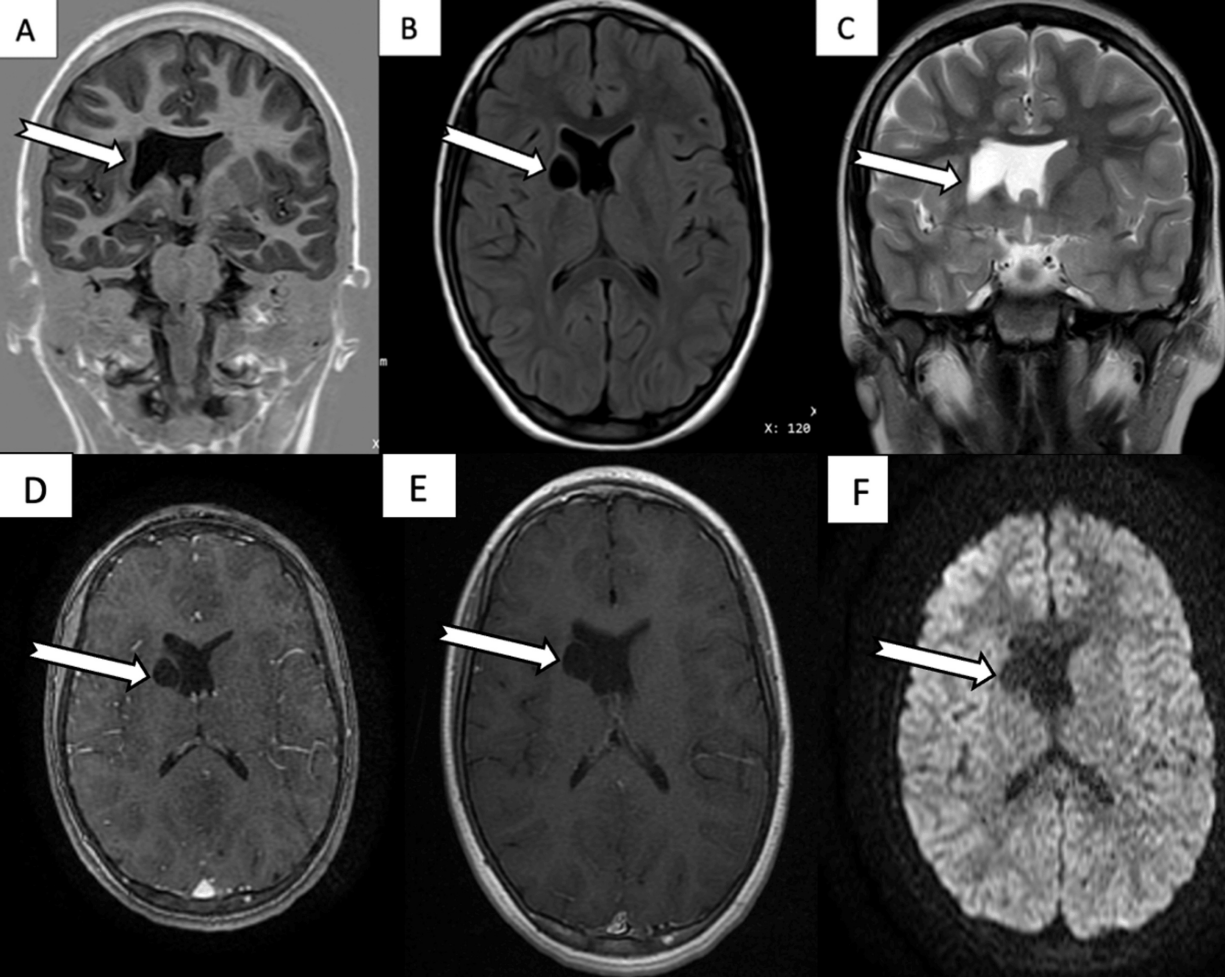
Brain MRI. (A) Coronal T1-weighted image showing a hypointense cystic lesion in the right periventricular region, adjacent to the head of the caudate nucleus and the anterior limb of the internal capsule. (B) Axial FLAIR image showing the lesion as hypointense. (C) Coronal T2-weighted image showing the lesion as hyperintense. (D, E) Axial post-contrast T1-weighted images showing no enhancement of the lesion or surrounding parenchyma. (F) DWI showing no diffusion restriction. MRI: magnetic resonance imaging; FLAIR: fluid-attenuated inversion recovery; DWI: diffusion-weighted imaging
